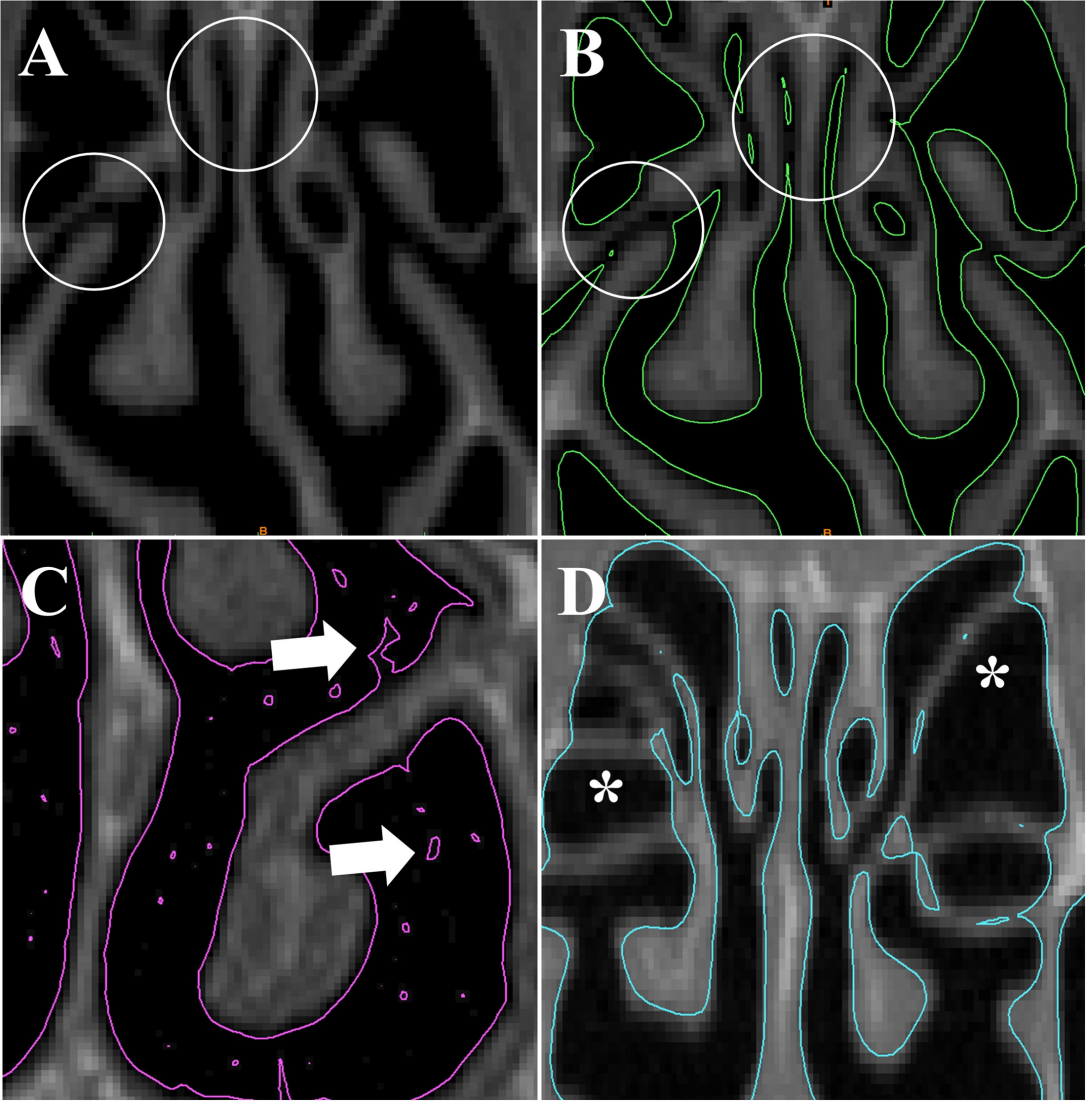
Common artifacts observed when segmenting the nasal cavity from CT scans. (A) Coronal CT of patient #2 shows a patent ostiomeatal complex and a patent olfactory cleft (circles). (B) Contours of 3D reconstruction created with thresholding range -1024HU to -950HU show discontinuities in the airspace (circles). (C) In patient #1, the 3D reconstruction created with the same thresholding range has surface irregularities and noise (arrows). (D) In patient #3, the 3D model created using the thresholding range -1024HU to -200HU did not segment the walls of the ethmoid sinuses correctly.
